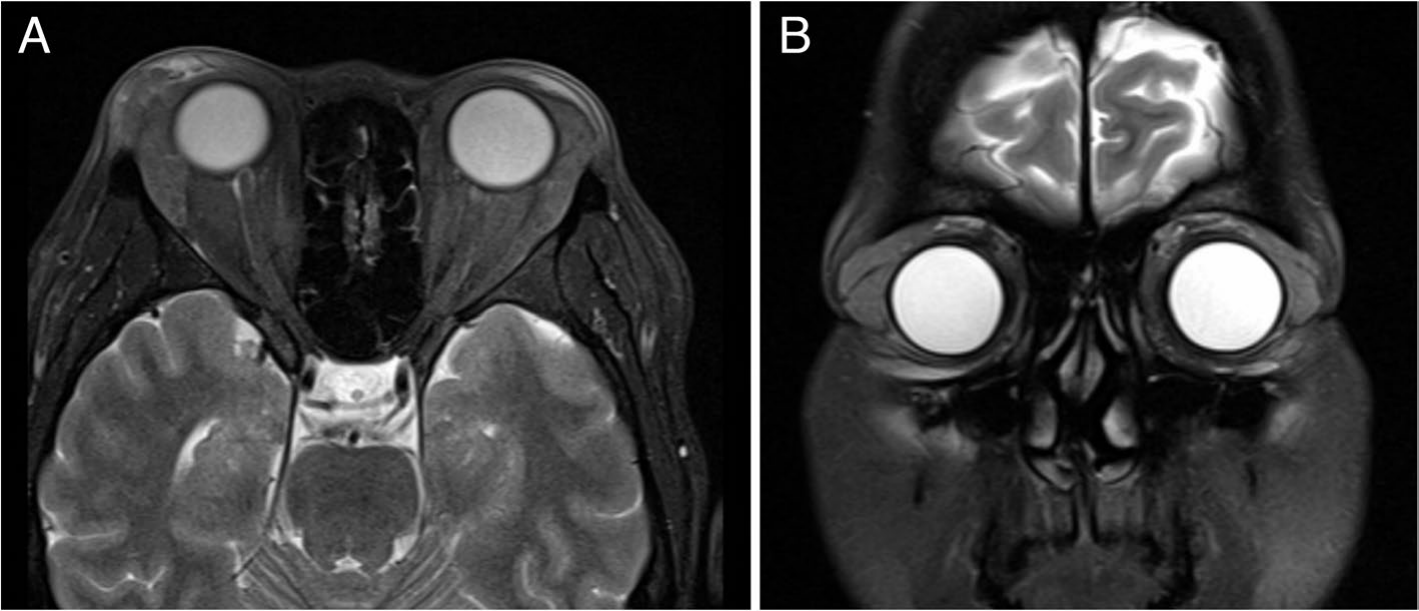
Axial (A) and coronal (B) T2-weighted MRI demonstrating diffuse enlargement of the lacrimal glands bilaterally with surrounding inflammatory changes. Lesions are softly heterogenous with subtle loculations and do not indent the globe. The lesion reaches far posteriorly in the orbit bilaterally, left greater than right
